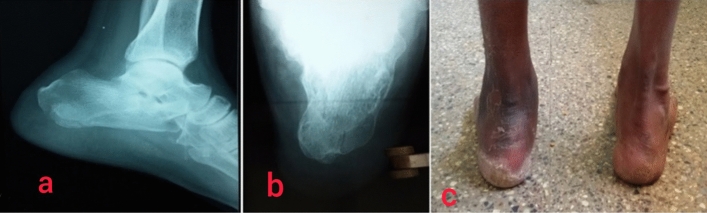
Complication after calcaneal fracture. (a,b) Radiographs showing calcaneal fracture with valgus deformation, (c) clinical photo of a patient with heel valgus deformity.
